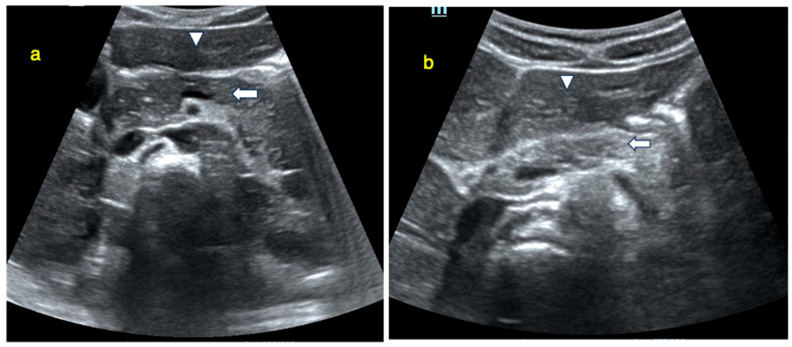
Ultrasound image showing pancreas echogenity compared to liver. (a): Ultrasound image showing pancreas (arrow) with equal echogenity compared to liver (arrowhead). (b): Ultrasound image showing hyperechoic pancreas (arrow) compared to liver (arrowhead).
